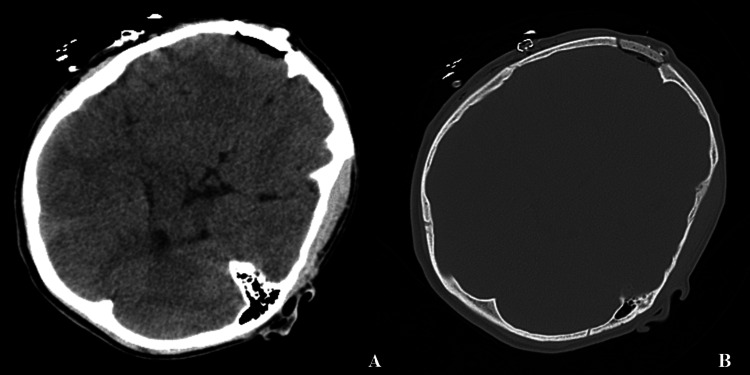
Postoperative computed tomography of the brain after total removal of the lesion and cranioplasty
